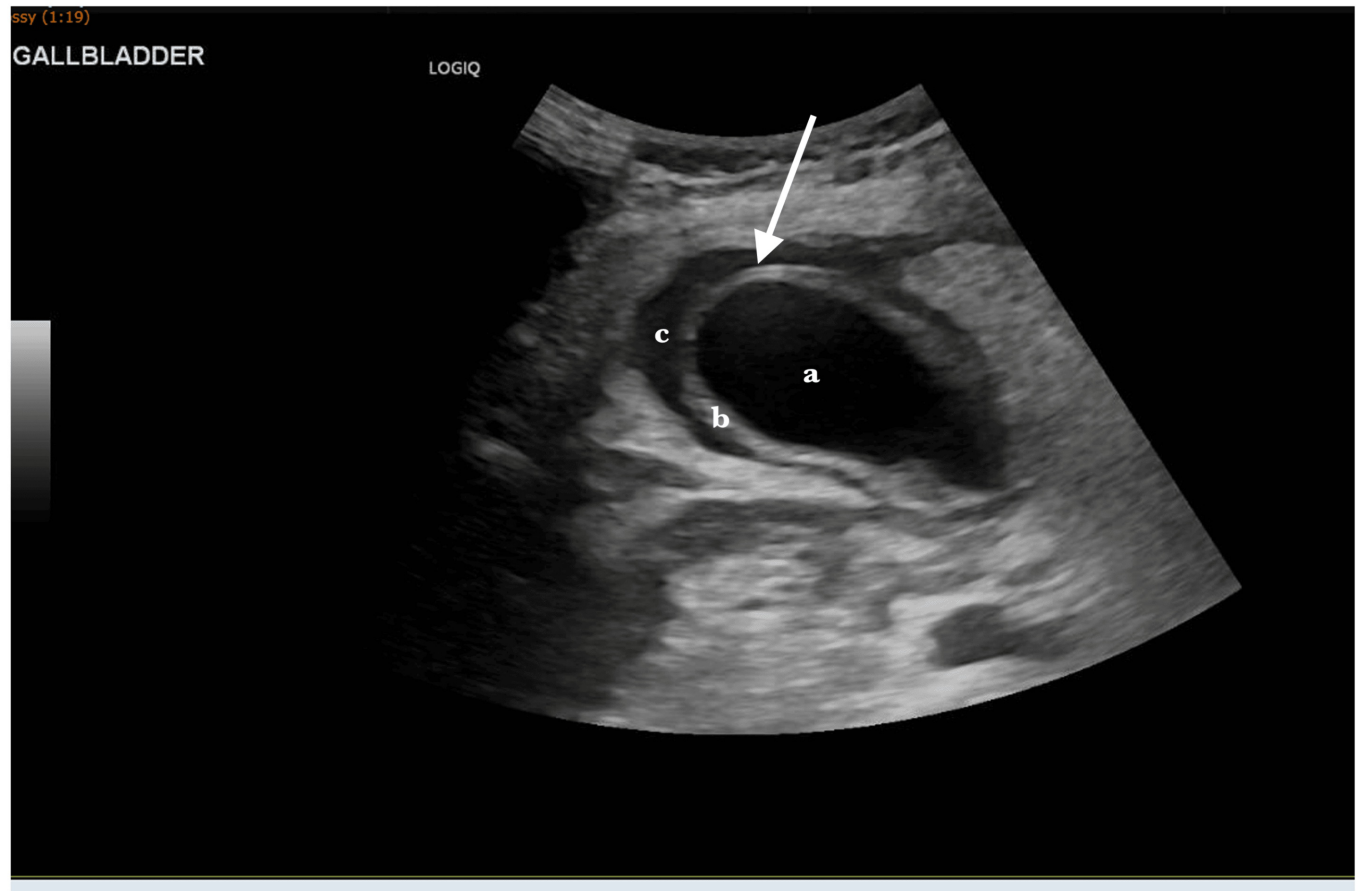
Abdominal ultrasound revealing gallbladder structures (arrow). Transabdominal ultrasound image demonstrating a markedly distended gallbladder (a) with diffuse wall thickening (b) and a hypoechoic rim of pericholecystic fluid (c), consistent with inflammatory changes. No echogenic foci or posterior acoustic shadowing were identified to suggest gallstones.
